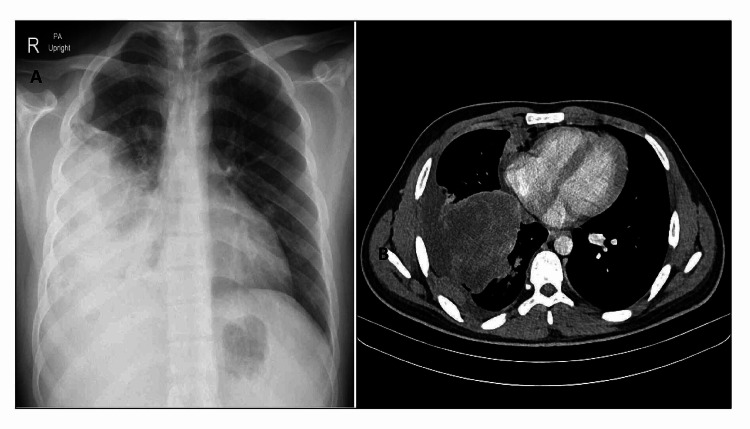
(A) Chest x-ray: right middle and lower lobe mass with right-sided pleural effusion. (B) Chest CT: right lower lobe mass with multiple smaller bilateral masses. CT, Computed tomography.
